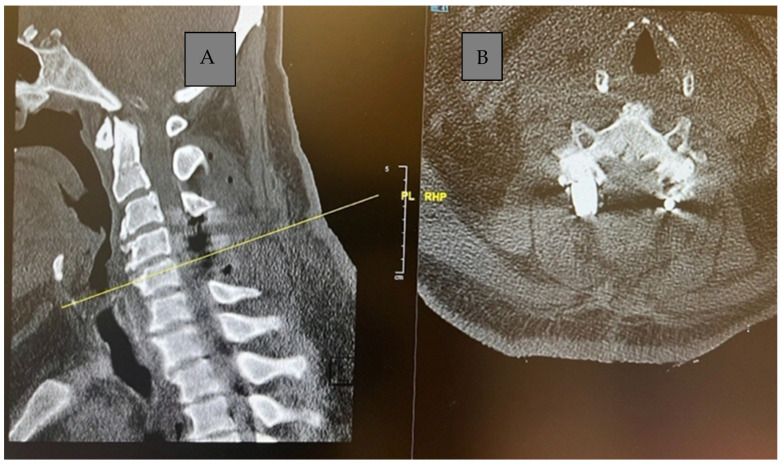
Postoperative CT scan of the cervical spine showing complete decompression at the cervical 4 and 5 levels. (A) Postop CT cervical spine sagittal view showing satisfactory decompression. (B) Postop CT cervical spine axial view showing posterior decompression and improvement in canal stenosis.
